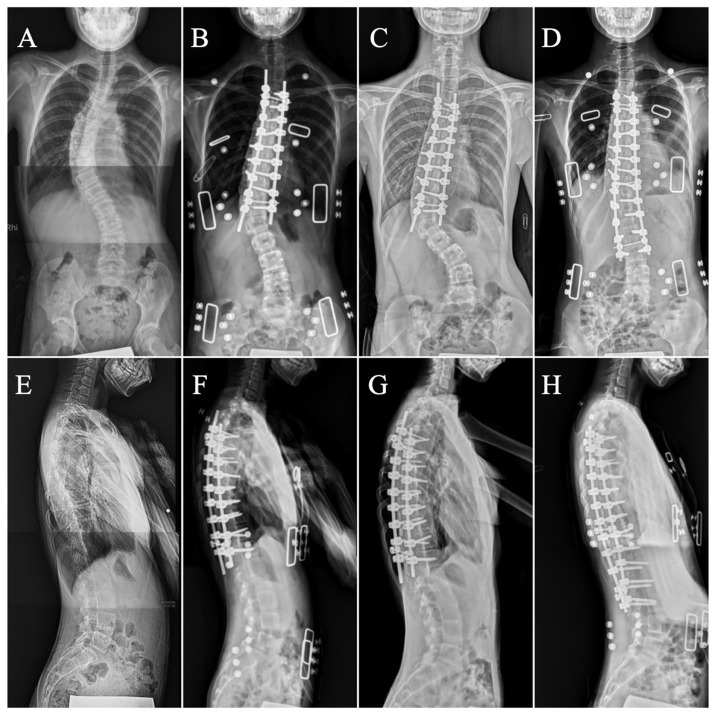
Postoperative decompensation of the lumbar curve and after revision surgery, (A) preoperative AP X-ray, (B) postoperative day 7, (C) postoperative decompensation year 3, (D) postrevision surgery day 7, (E) preoperative day 5 lateral X-ray, (F) postoperative day 7, (G) postoperative progression year 3, and (H) post-revision surgery day 7.
